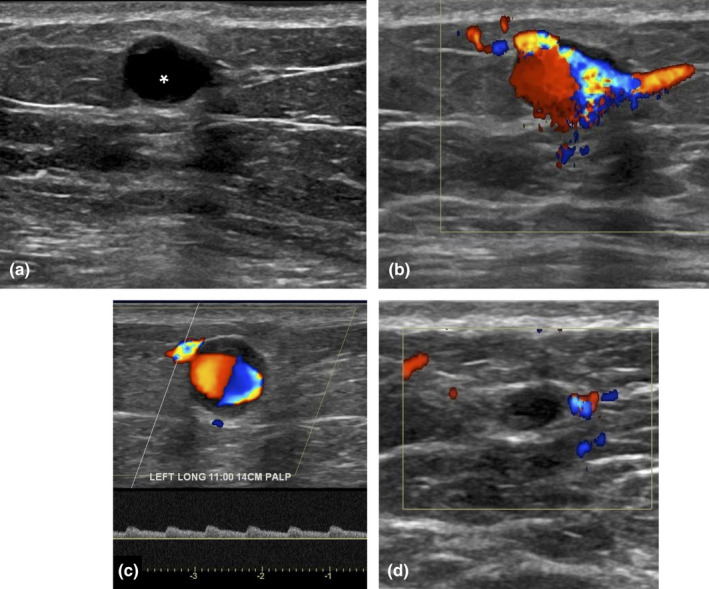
41‐year‐old woman with no prior history of trauma or intervention presented with a palpable left breast lump. Targeted ultrasound showed a 1.0 cm anechoic cystic structure (asterisk) at 11 o'clock 14 cm from the nipple (a), with turbulent flow colour with some mural thrombus (b). Spectral Doppler of the feeding vessel demonstrates a low resistive arterial waveform (c) and to‐and‐fro flow was not seen. This did not have the typical appearance of a pseudoaneurysm, but rather a true aneurysm. Given this, thrombin injection was deferred, and surgery consultation was recommended. Breast and vascular surgery agreed on short‐interval follow‐up given the mild symptoms. This patient returned 5 months later, and the true aneurysm was smaller in size and had completely thrombosed (d).
